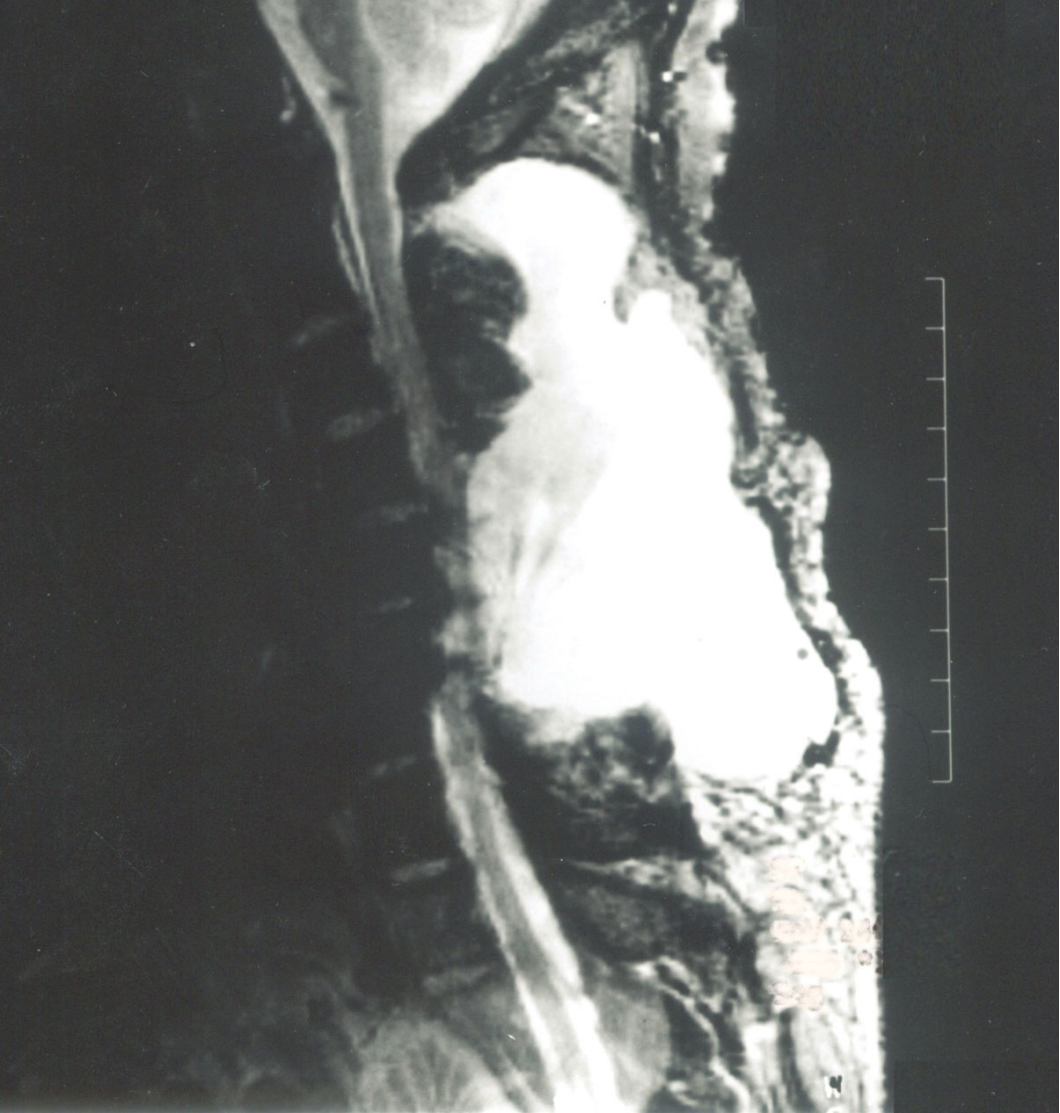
A giant pseudomeningocele noted after surgery for C5-C6 recurrent disk herniation. A 53-year-old male underwent cervical laminectomy and discectomy for C5--C6 recurrent disk herniation. After surgery, a giant cervical pseudomeningocele (length, 11 cm; width, 5 cm; depth, 5 cm) was noted on T2-weighted MRI.
